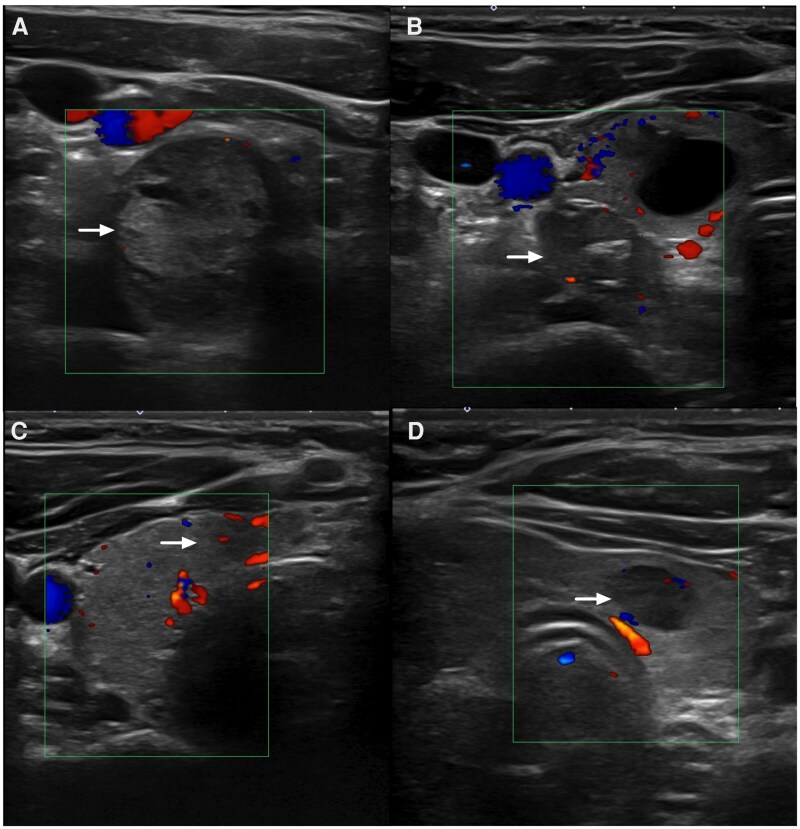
Ultrasound images of described thyroid nodules are depicted, with white arrows identifying the nodule and color Doppler present in each panel. (A) Right lower pole nodule. (B) Right midpole nodule. (C). Thyroid isthmus nodule. (D). Residual left lobe nodule.
